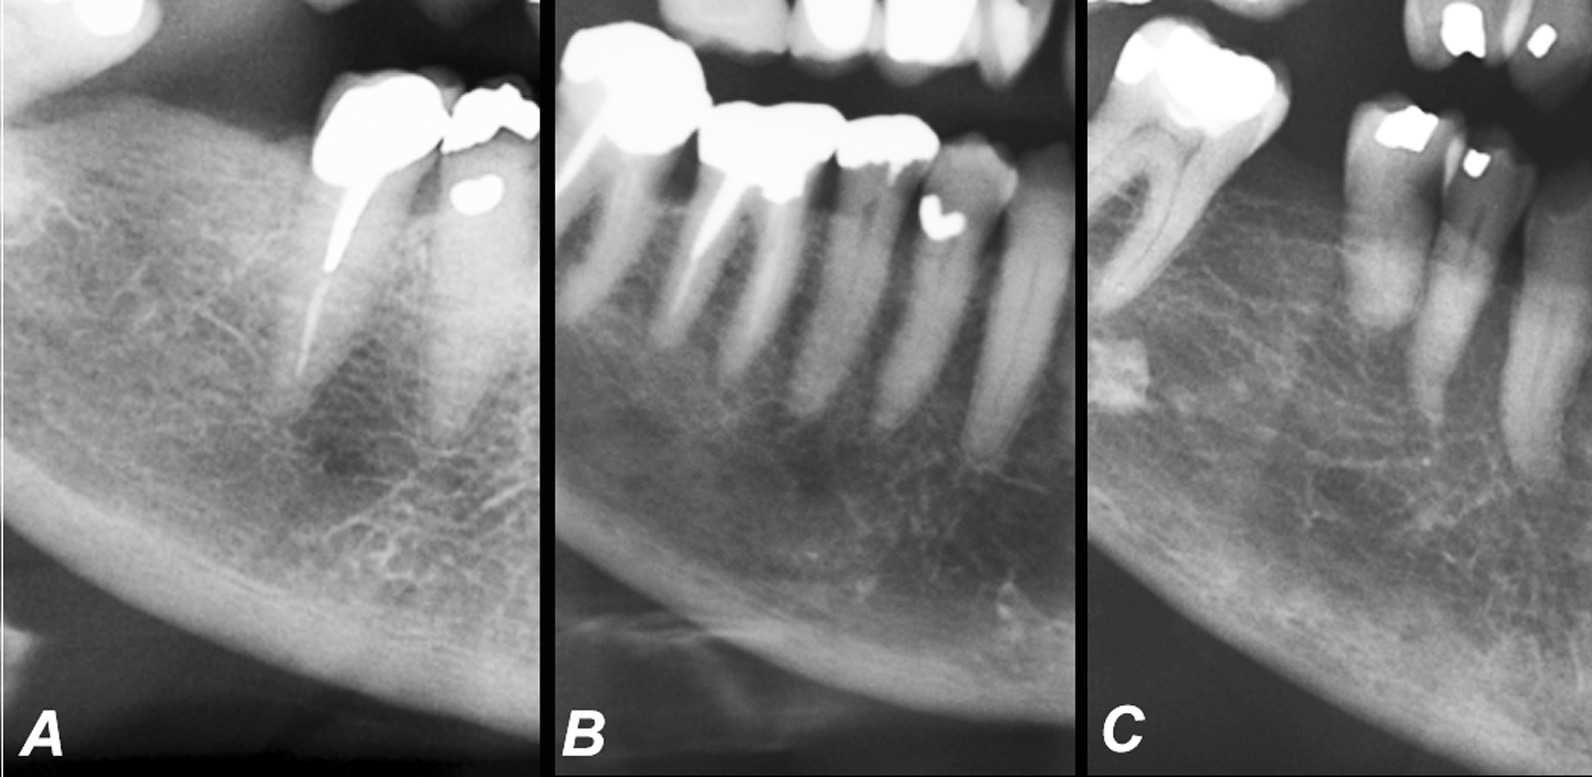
Reference panoramic radiographs for mandibular cortical index (MCI). a A normal cortex (MCI-1) with an even endosteal margin. b A moderately eroded cortex (MCI-2) with semilunar defects. c A severely eroded cortex (MCI-3) with heavy endosteal cortical porosities
